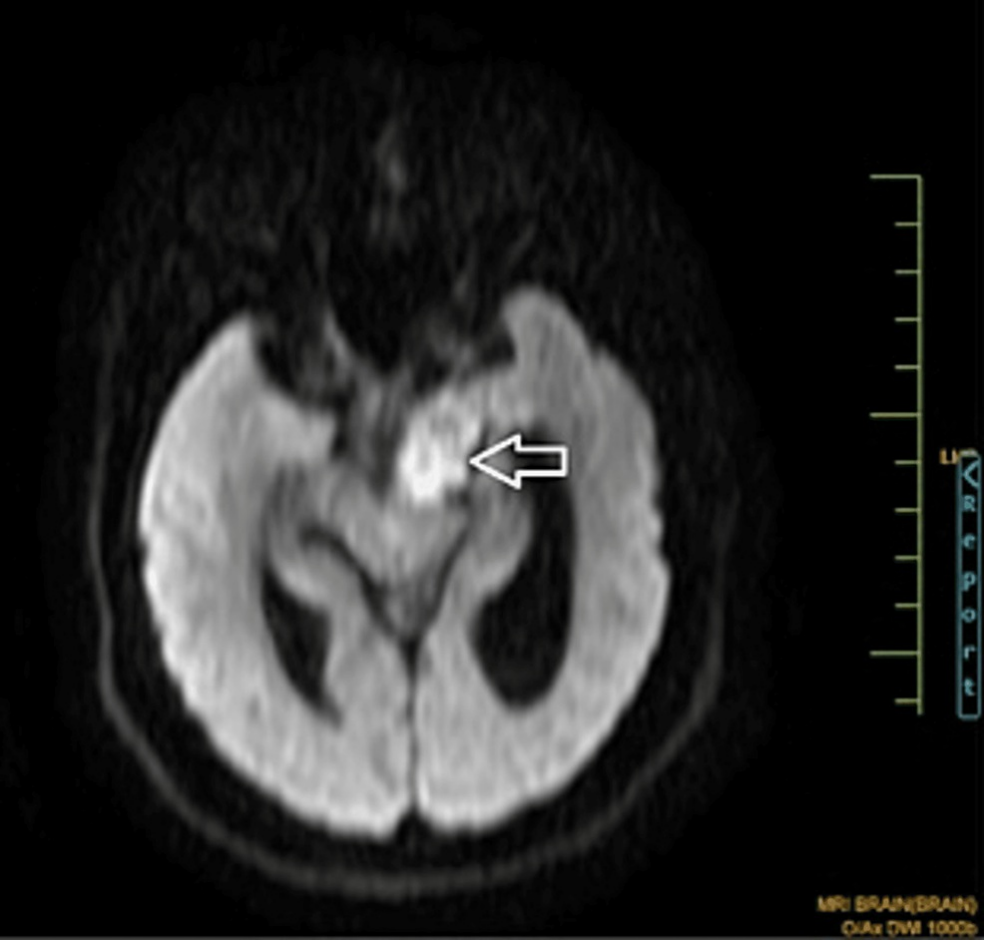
An axial MR DWI showing restricted diffusion Self-clicked image MR: magnetic resonance; DWI: diffusion-weighted imaging
